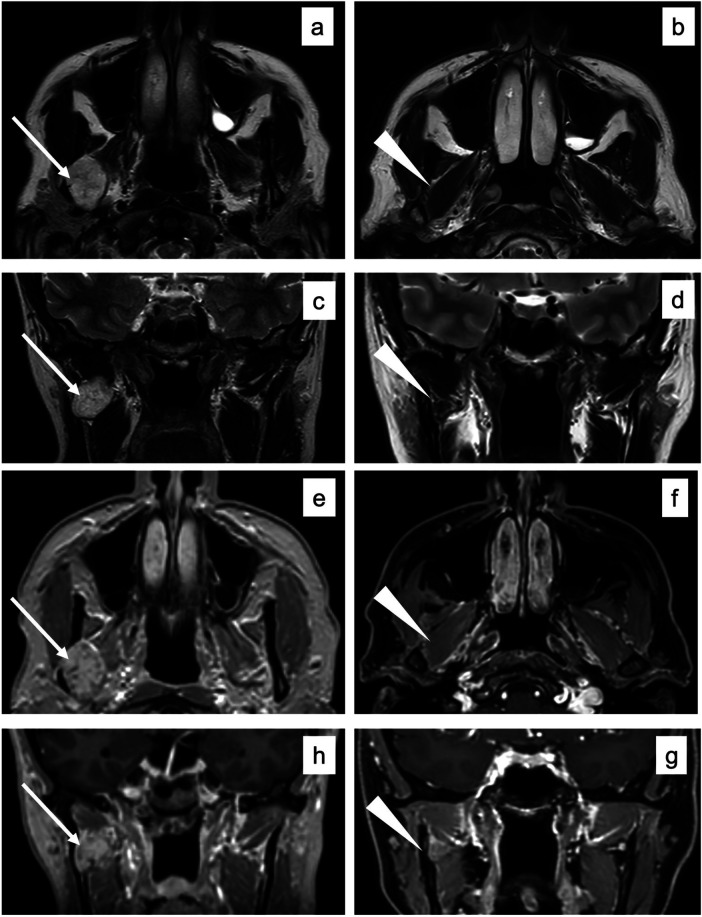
Axial (a,b) and coronal (c,d) magnetic resonance T2-weighted imaging without fat suppression and T1-weighted axial (e,g), without fat suppression, and coronal (f,h), with fat suppression. In presurgical images, a hyperintense round margin hypervascular mass is evident in the masticator space, located posteriorly to the distal part of the temporal muscle, medially to the mandibular ramus, and laterally to the lateral pterygoid muscle (a,c,e,g) arrows). In postsurgical images, the mass is completely removed, with restitutio ad integrum of the masticator space and its structures (b,d,f,g, arrowheads).
